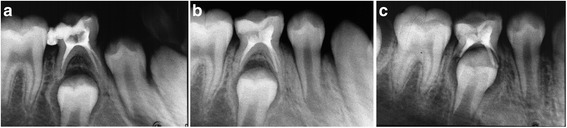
Twelve months follow-up of ZOE. a Radiograph of 84 treated with ZOE taken immediately postoperatively showing adequate filled. b Radiograph taken 6 months postoperatively showing slower resorption rate of filling material than roots. c Radiograph taken 12 months postoperatively showing remaining of filling material
